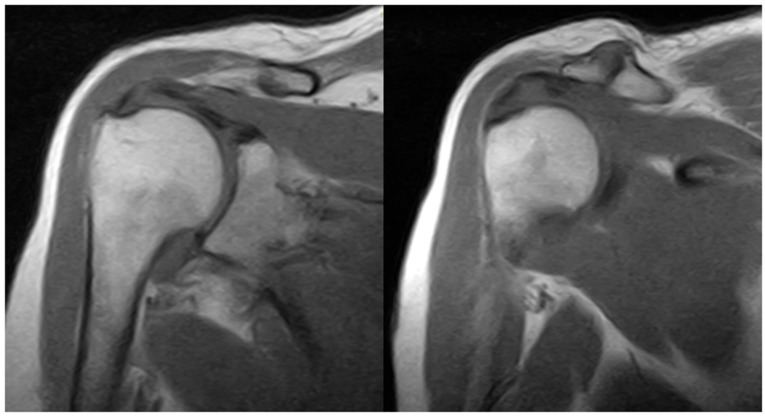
Shoulder MRI showing mild acromioclavicular arthrosis and severe supraspinatus tendinosis with intratendinous microinjuries and oedema.
